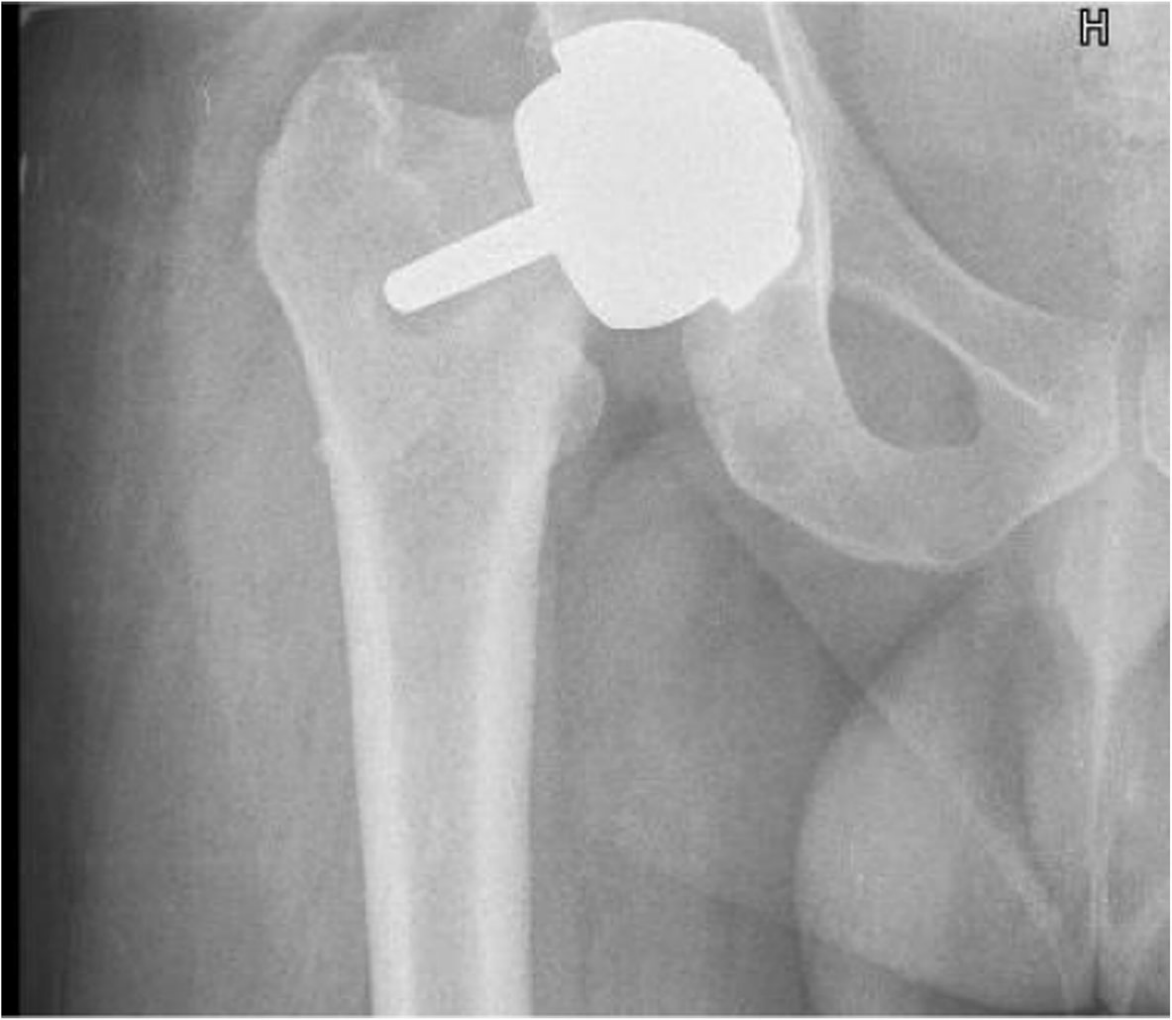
Radiographic image showing radiolucency in one patient
